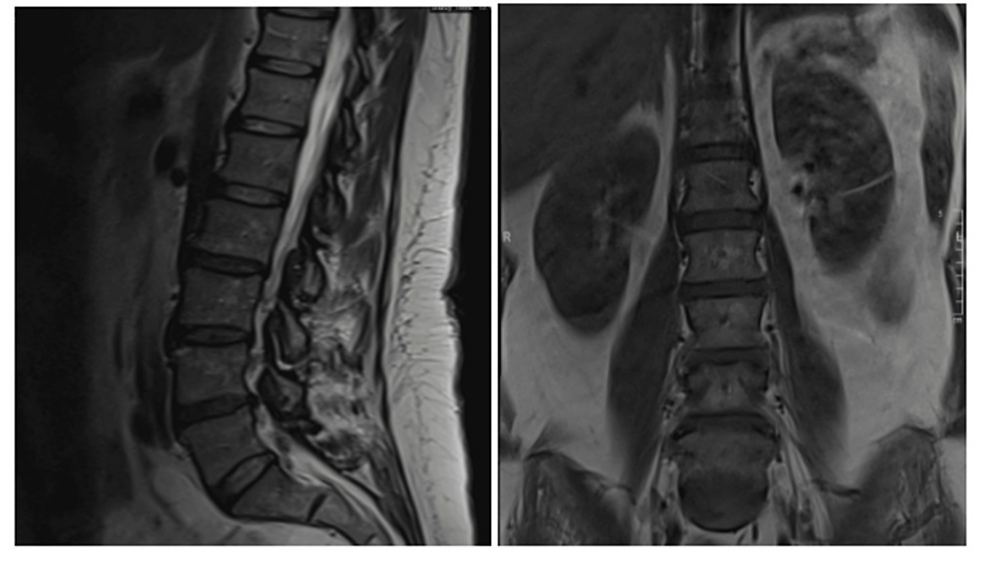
MRI of Lumbar Spine Without Contrast Imaging demonstrating mild lumbar spondylosis, moderate disc degeneration at L4-L5, mild disc degeneration at L2-L3 and L3-L4, no traumatic injury, fracture, subluxation, or suggestion of acute process of infection. MRI, magnetic resonance imaging.
